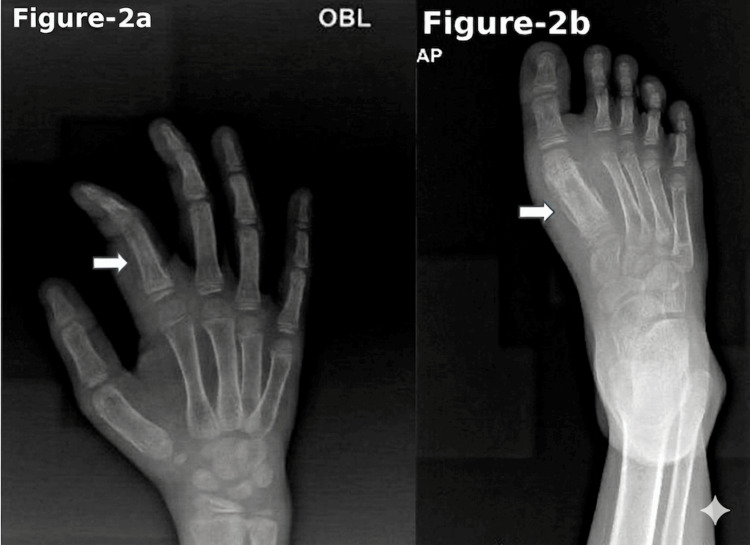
Clinical and radiographic features of the right foot (a) Plain radiograph (anteroposterior view) of the right hand revealing an expansile, "ballooned" lytic lesion (spina ventosa) of the proximal phalanx of the index finger with cortical thinning and medullary widening. (b) Oblique radiograph of the right foot revealing a well-defined, expansile lytic lesion involving the first metatarsal bone. The image shows significant cortical thinning and trabecular destruction without joint space involvement, consistent with tuberculous dactylitis.
